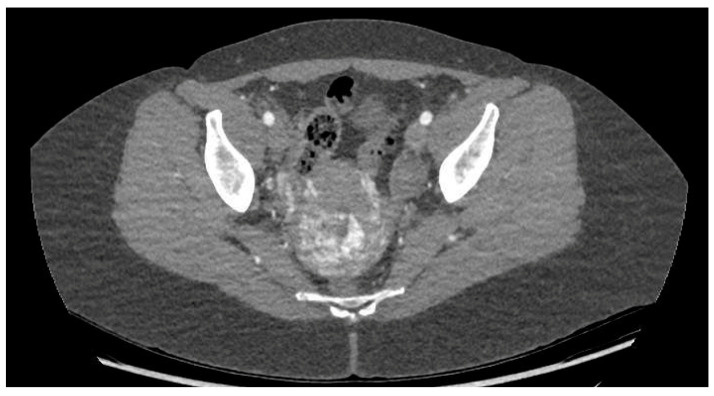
Cross-sectional pelvic CT angiography.
